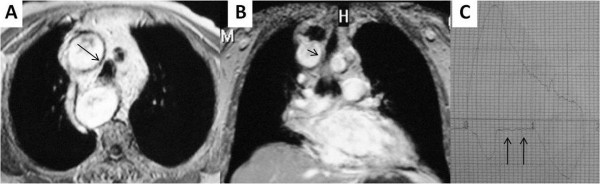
Thoracic MRI and spirometry images; right sided arcus aorta with tracheal compression as seen on thoracic MRI (2A, 2B, black arrows). Spirometry showed a plateau on flow-volume curves throughout inhalation (2C).
